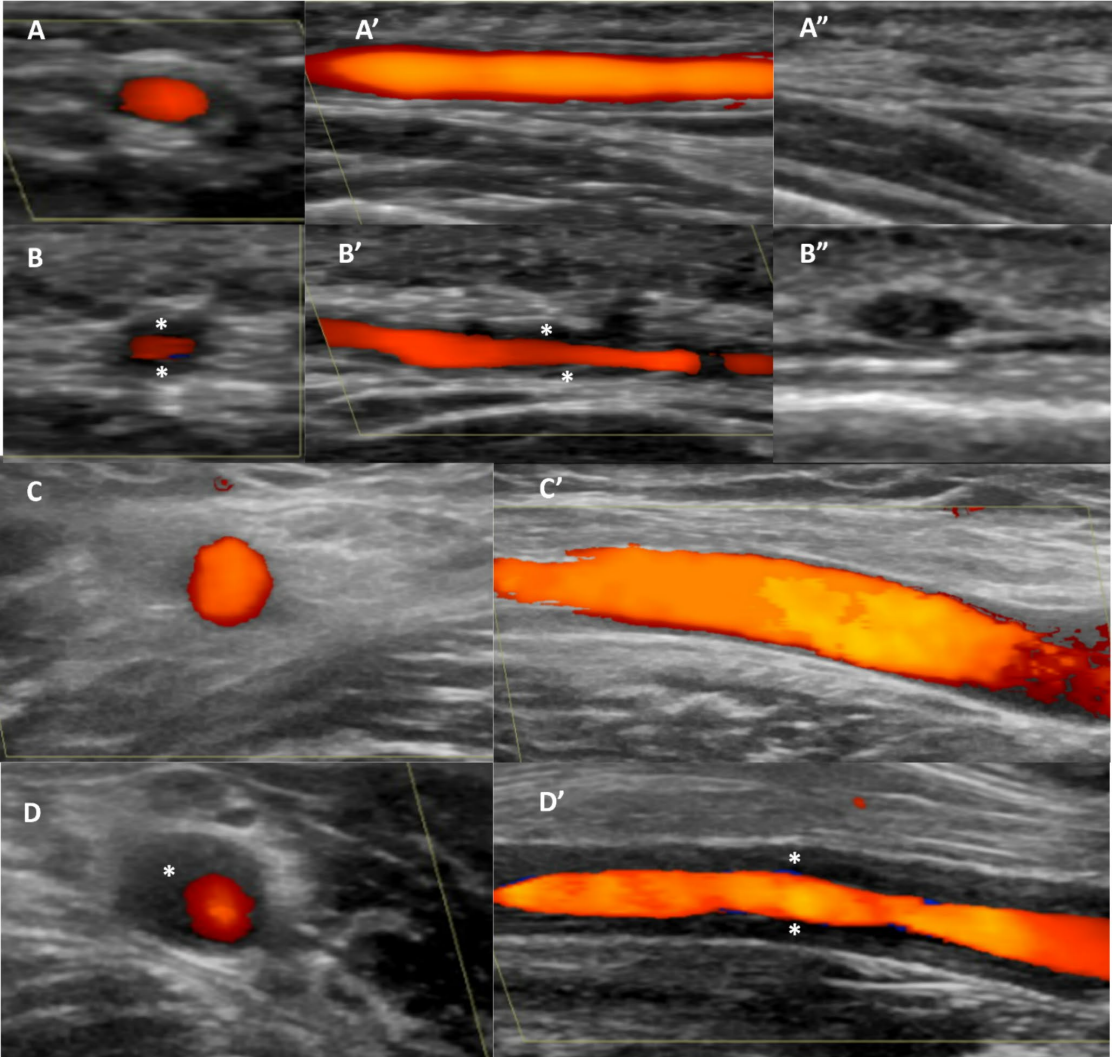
Ultrasound in the assessment of vessels (large vessel vasculitis). A shows the ultrasound scan of the superficial temporal artery in a healthy subject, demonstrating normal vessel wall thickness in both transverse (A) and longitudinal (A’) views. The vessel wall disappears upon probe compression (A’’). The figure also includes images of the superficial temporal artery in a patient with giant cell arteritis, displaying the halo sign in transverse (B) and longitudinal (B’) views (marked with asterisks). In B’’, the thickened arterial wall remains visible despite external compression, indicating the compression sign. C presents a normal axillary artery in transverse (C) and longitudinal (C’) views in a healthy subject. D and D’ display the halo sign in the axillary artery in transverse and longitudinal views, respectively, in a patient with Takayasu disease
